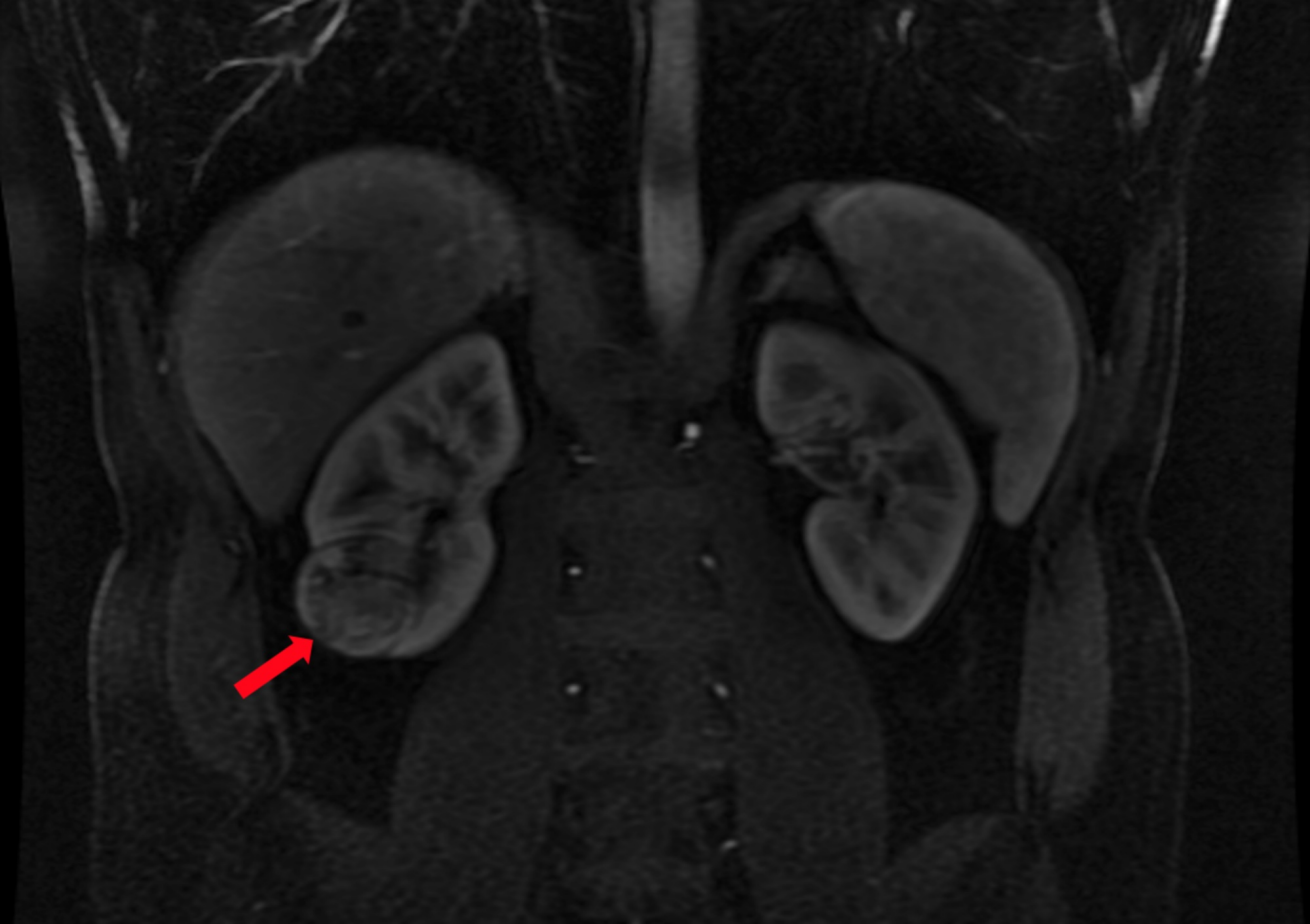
MRI showing image of right lower pole renal mass (red arrow)
